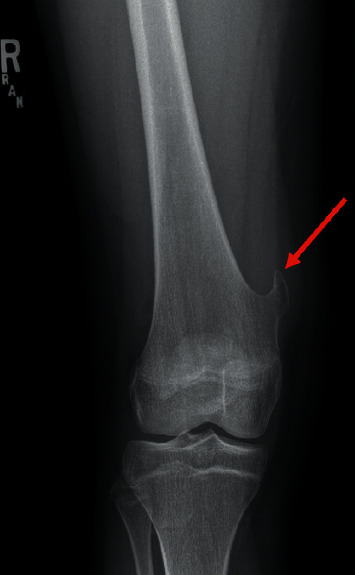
An anterior posterior radiograph of the patient's right knee with limited view of the femur showing the hook-shaped osteochondroma (red arrow).
